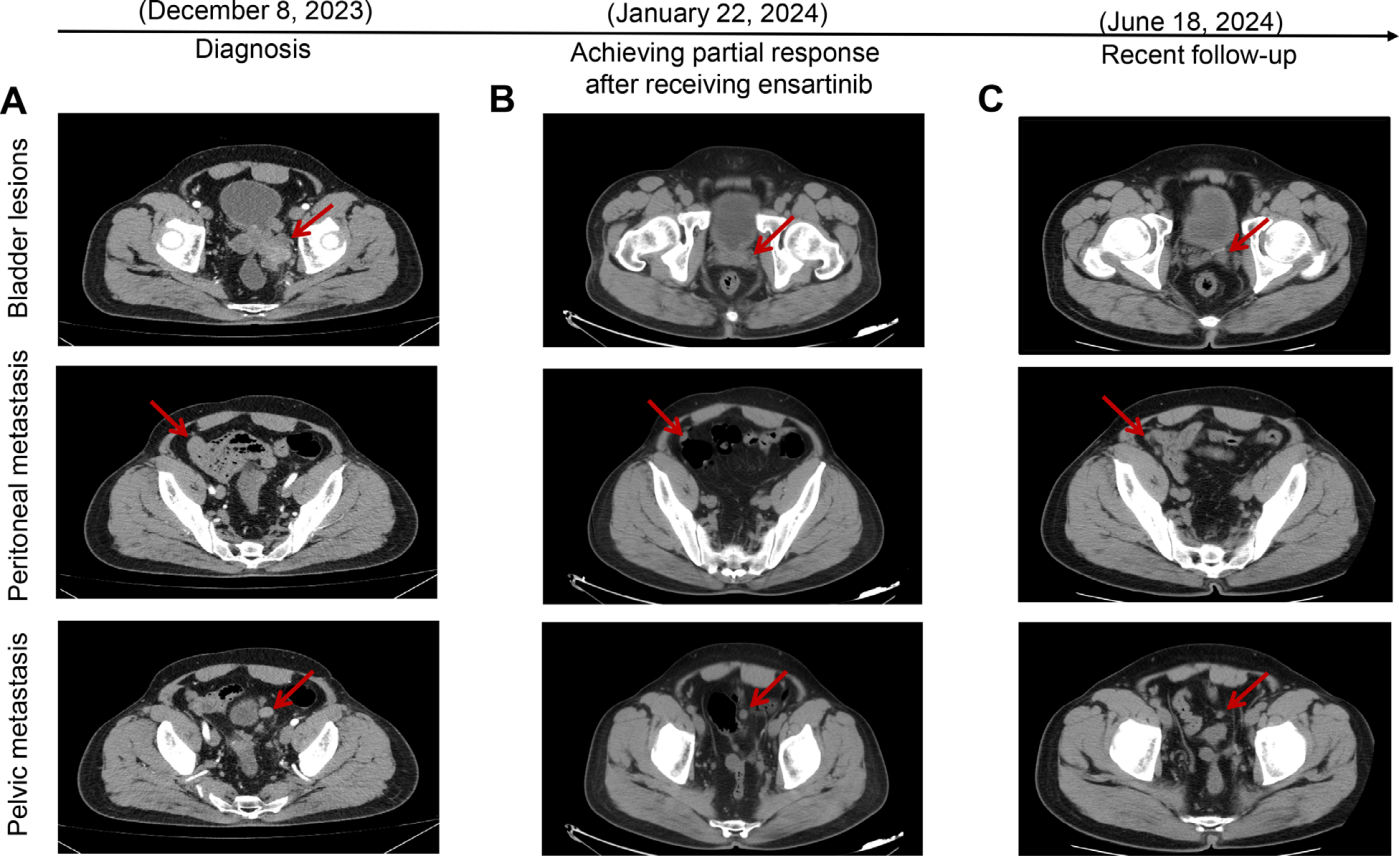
Target lesions of the patient with IMTUB by CT scans. (A) CT scans indicated space-occupying lesions of the bladder, with peritoneal and pelvic metastasis. (B) CT scans revealed tumors had decreased in size, achieving partial response after receiving ensartinib treatment. (C) Recent follow-up CT scans showed that the patient maintained partial response. IMTUB, inflammatory myofibroblastic tumor of the urinary bladder; CT, computed tomography.
